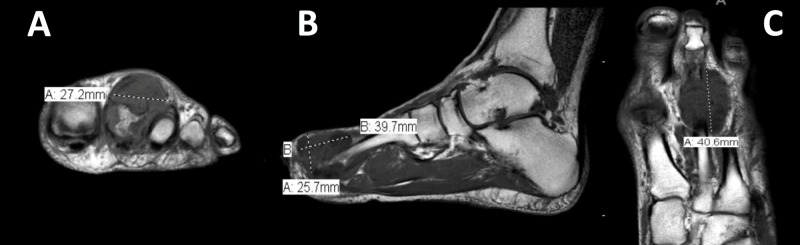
Pre-operative magnetic resonance imaging (MRI) of the left foot without contrast: (A) coronal, (B) sagittal, and (C) axial images at the level of the lesion with measurements
